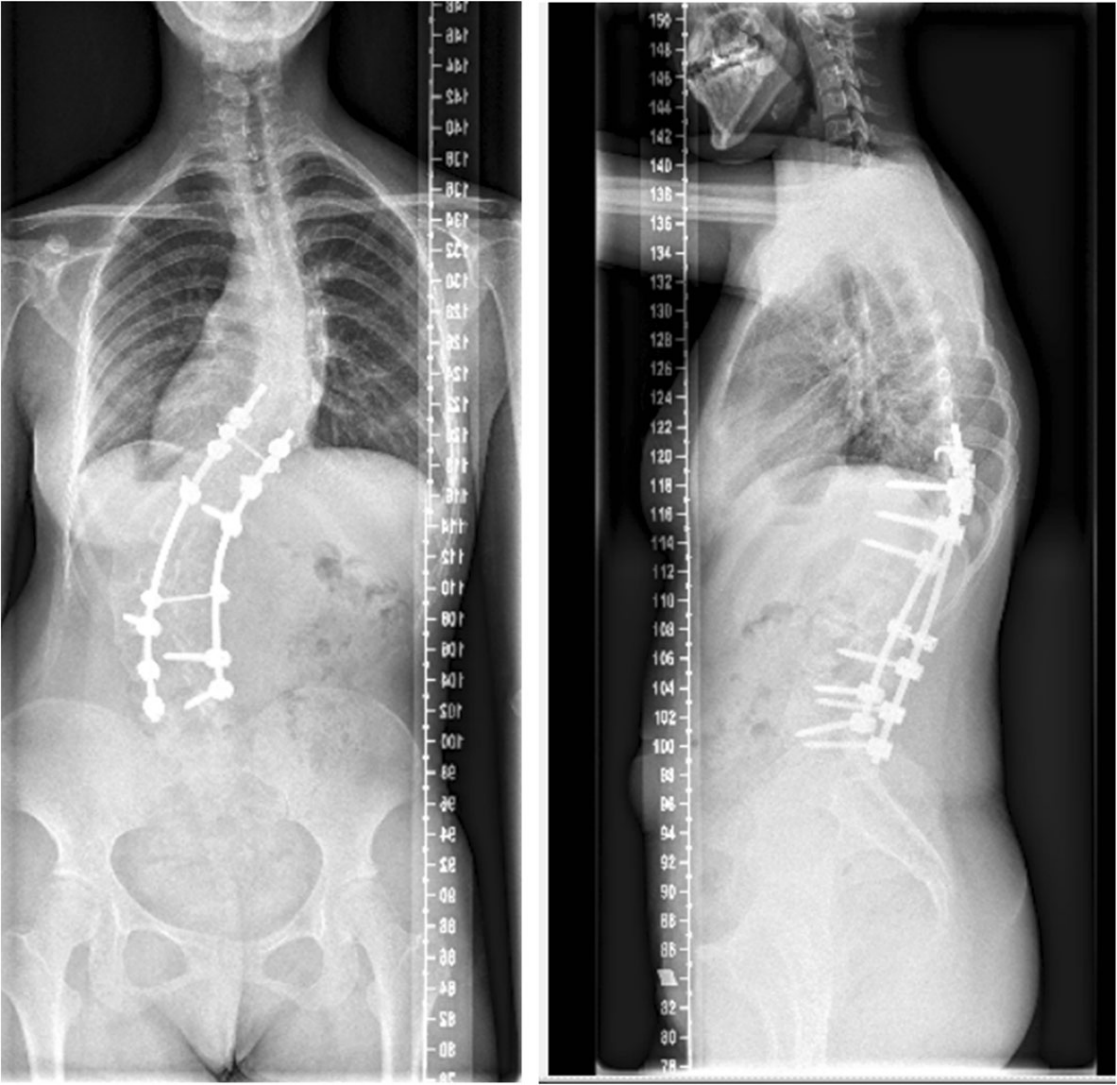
Standing anteroposterior and lateral radiographs of 3 months after operation
